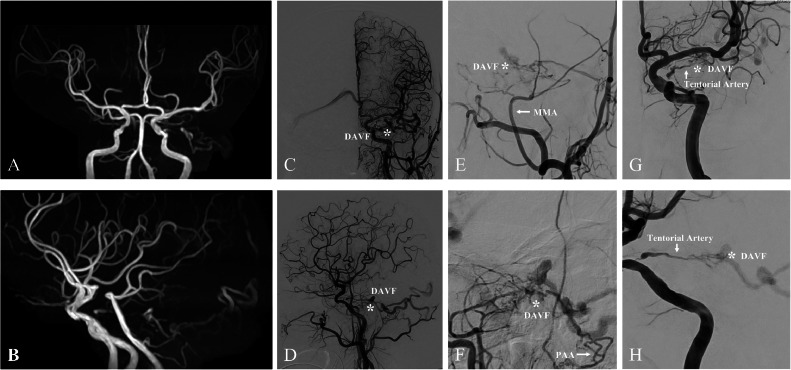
The shunt point of DAVF is indicated (asterisk). (A,B) Magnetic resonance imaging (MRI) reveals a left tentorial DAVF. (C,D) Digital subtraction angiography (DSA) of the left common carotid artery showing that the tentorial DAVF (asterisk) is fed from the left tentorial, left middle meningeal, and left posterior auricular arteries. A shunt pouch is formed in the left petrotentorial location. Drainage occurs via arterialization of the left petrosal vein, forms a varix, and drains into the confluence of the right transverse sinus. (E,F) DSA of the left external carotid artery showing that the middle meningeal artery (MMA) and posterior auricular artery (PAA) are tortuous and inaccessible near the shunt point of DAVF (asterisk). (G,H) DSA of the left internal carotid artery showing that the tentorial artery is a relatively straight and well-dilated vessel to the DAVF (asterisk), but the origin of the meningohypophyseal trunk (MHT) is highly stenotic.
